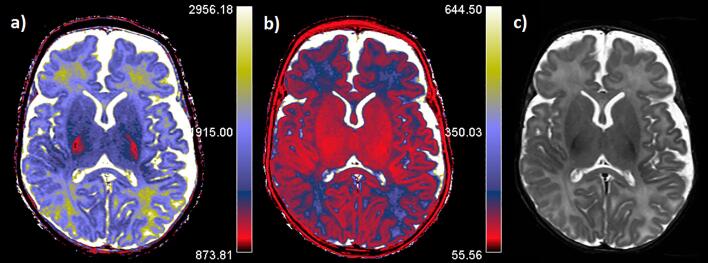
Representative sample of synthetic MRI-based T1 (a) and T2 (b) relaxation time maps. (c) Synthetic reconstructed T2 STIR image is shown at the same level.
